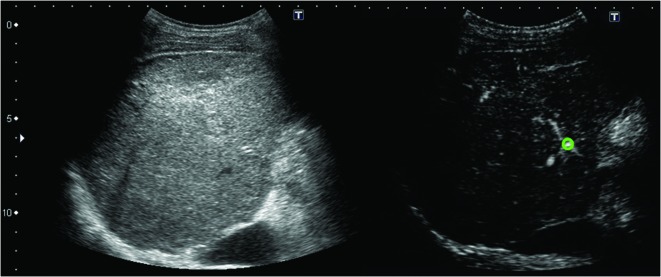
Arrival time parametric imaging (At-PI): Left, B-mode ultrasonography (US) image; right, a Sonazoid-enhanced US image. The region of interest (green circle) was set on the large hepatic artery branch in the right hepatic hilum.
